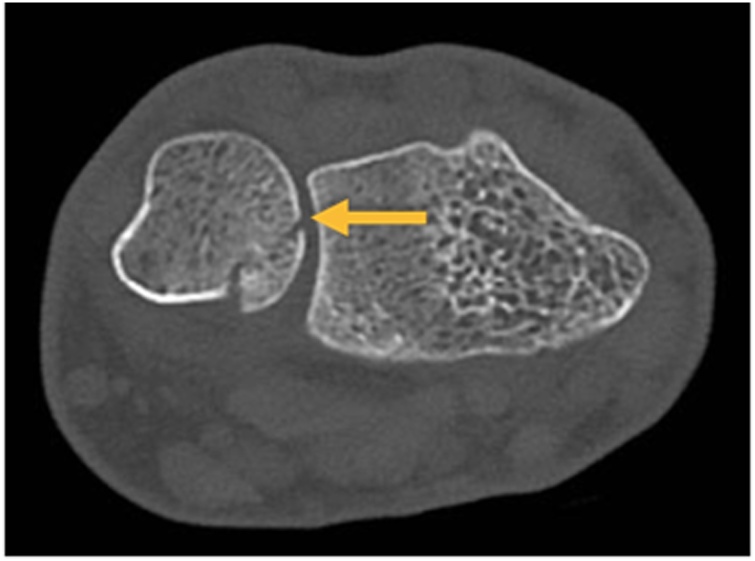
CT scan of the patient`s left wrist (axial view) showing an undisplaced Fracture of the ulna styloid (arrow).
